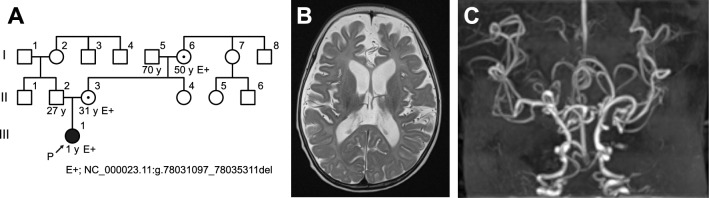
Clinical characteristics of the patient. (A) A family tree. Samples from individuals I-5, I-6, II-2, II-3, and III-1 were collected. (B) T2-weighted MR imaging showed diffuse atrophy of the cerebrum and delayed myelination in the splenium of the corpus callosum. (C) MR angiography showing intracranial tortuous arteries characteristic of Menkes disease.
